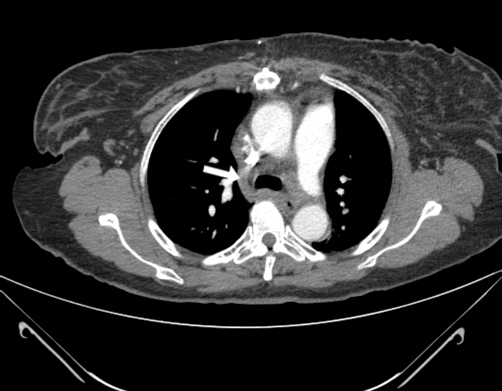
CT scan with contrast shows patent SVC at the site of previous stenosis after 2 months.
